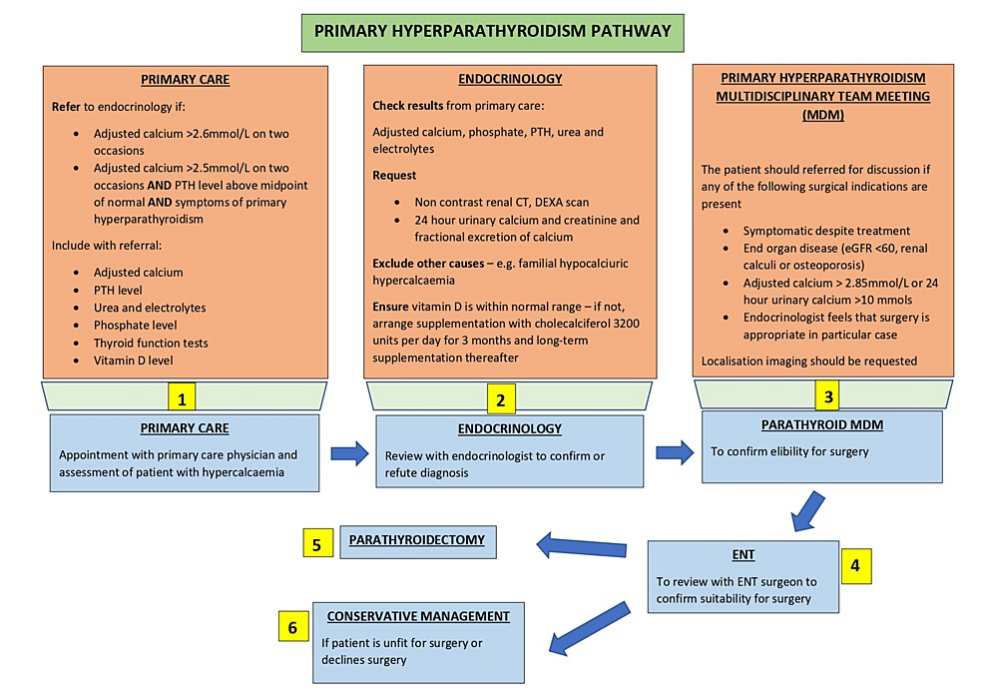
New proposed primary hyperparathyroidism management pathway DEXA, dual x-ray absorptiometry; eGFR, estimated glomerular filtration rate; BMI, body mass index; PTH, parathyroid hormone
